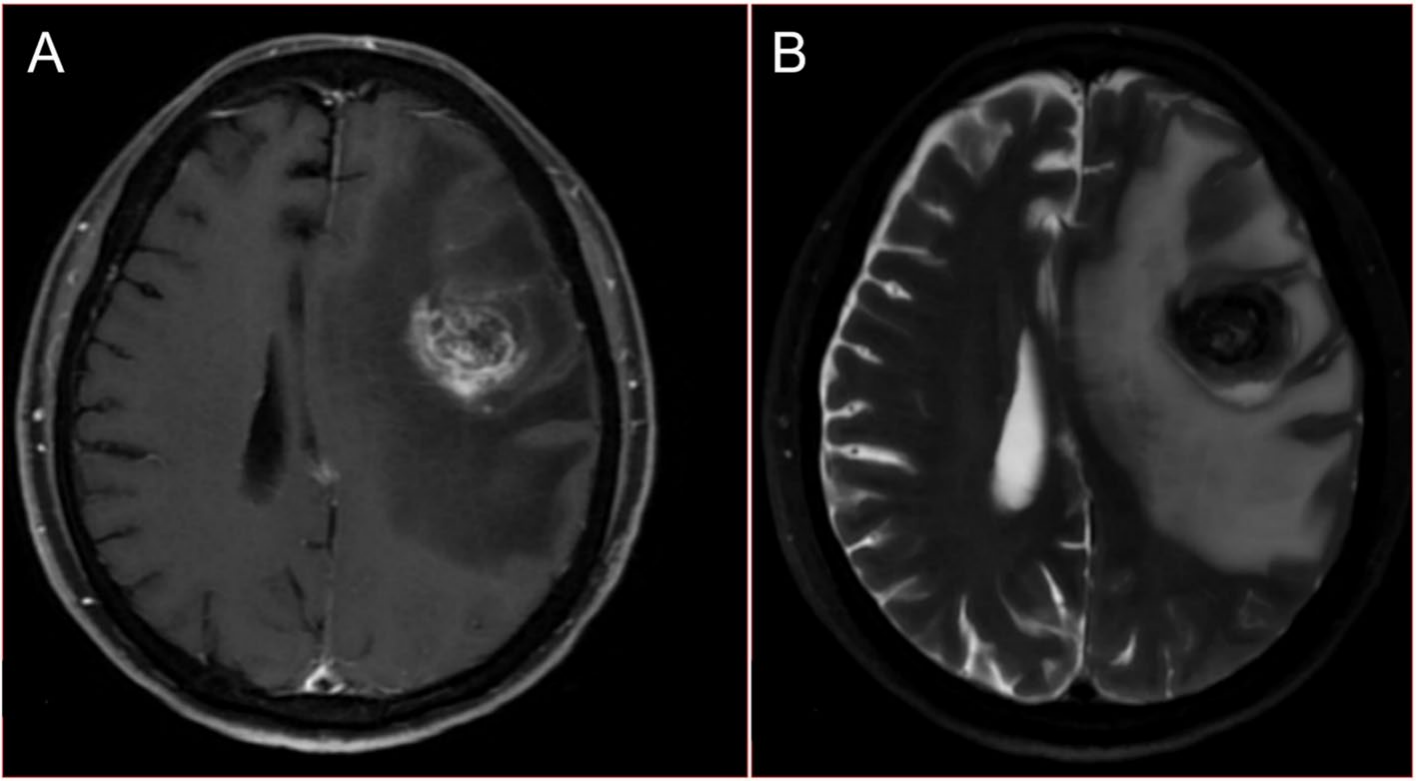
Magnetic resonance images of the head with the patient at admission. (A) Contrast enhanced T1WI. (B) T2WI.
